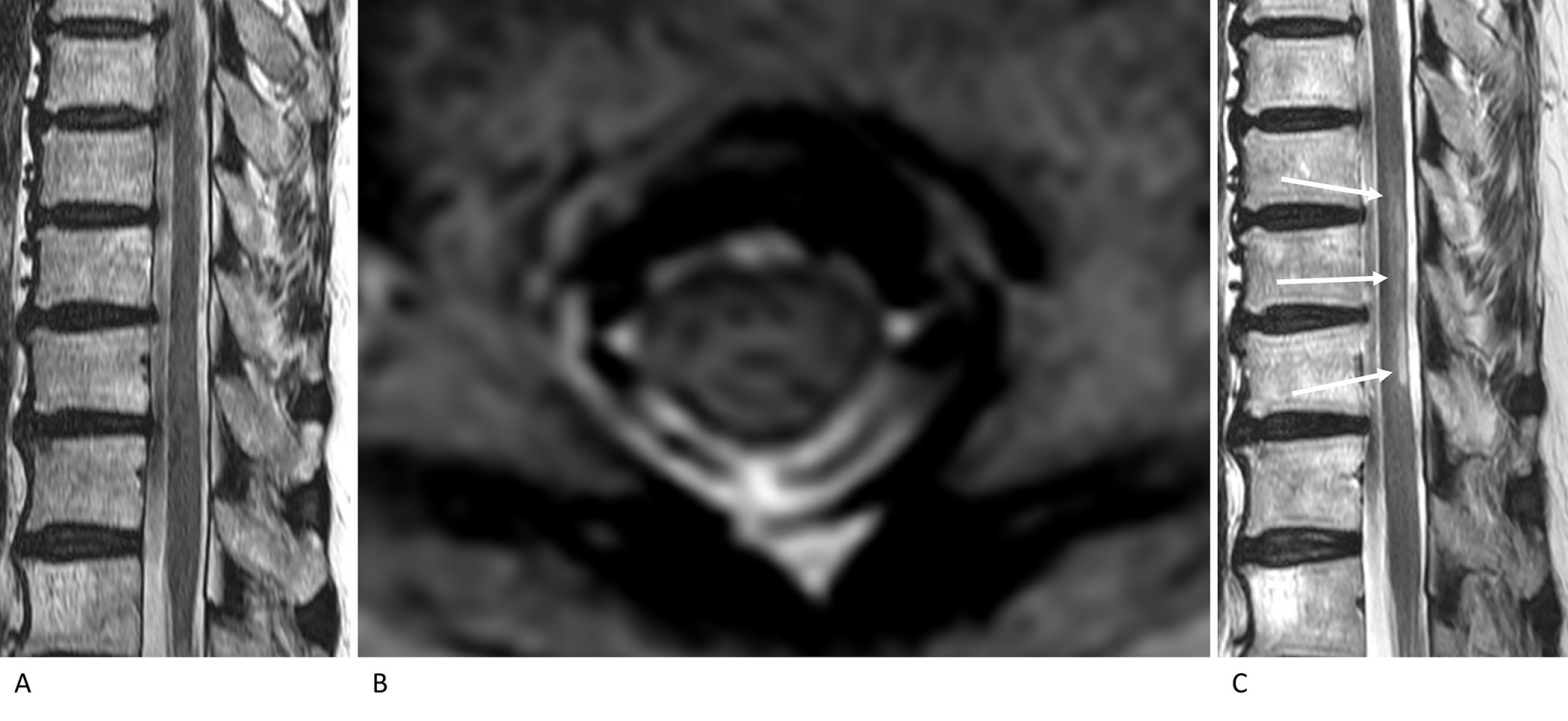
T2-weighted MRI of the lower thoracic spine in a patient with spinal cord infarction (80-year-old woman, patient number 9 in Table 4). On sagittal (A) and axial (B) images obtained between 6 and 12 h after symptom onset, changes in the cord signal are not obvious. The authors evaluated the confidence score to be 2. Follow-up MRI (C) performed between 24 and 72 h after symptom onset shows distinct hyperintensity in the dorsal spinal cord (arrows). The authors evaluated the confidence score to be 4.
